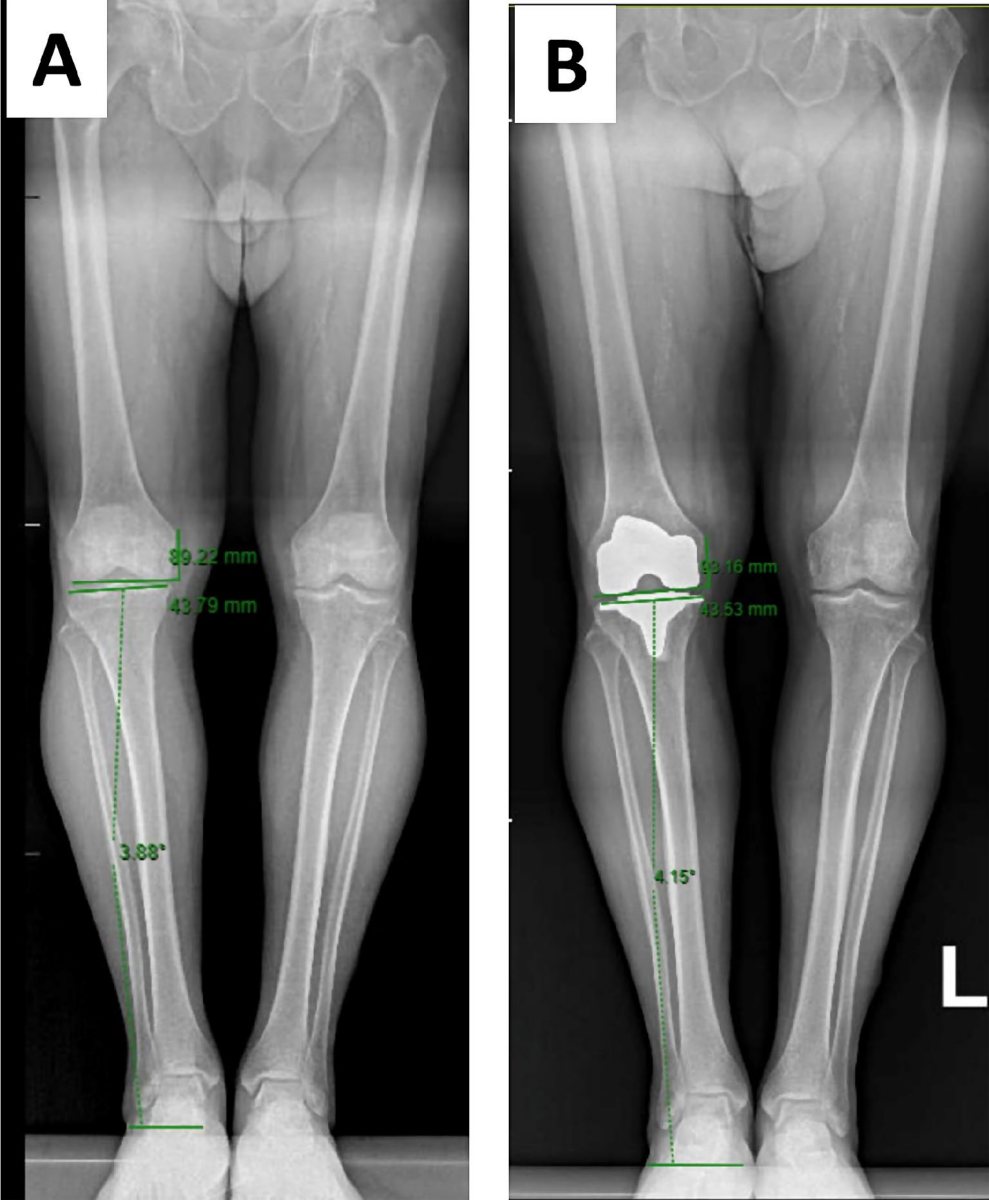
Preoperative (A) and postoperative (B) full-length standing AP radiographs with joint line obliquity and joint line height restoration measurements
